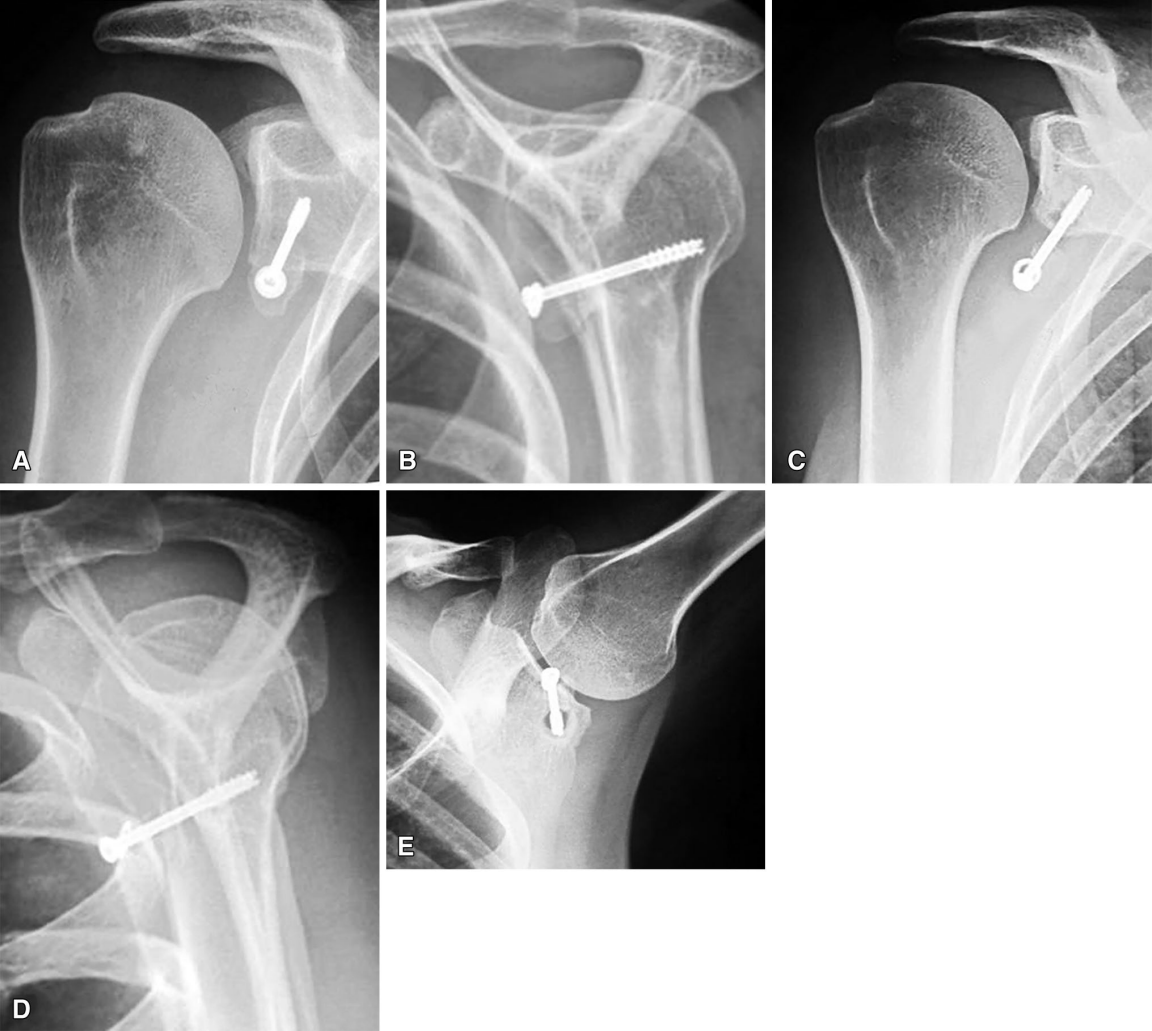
An example of coracoid graft nonunion is shown. Early postoperative (A) AP and (B) lateral radiographs demonstrate good positioning of the bone block but eccentric positioning of the screw in the bone block. (C) AP, (D) lateral, and (E) axillary radiographs at 3 years’ followup demonstrate loss of fixation and nonunion of the graft with screw pullout and a chamber of osteolysis. This patient was a 40-year-old woman and a heavy smoker.
